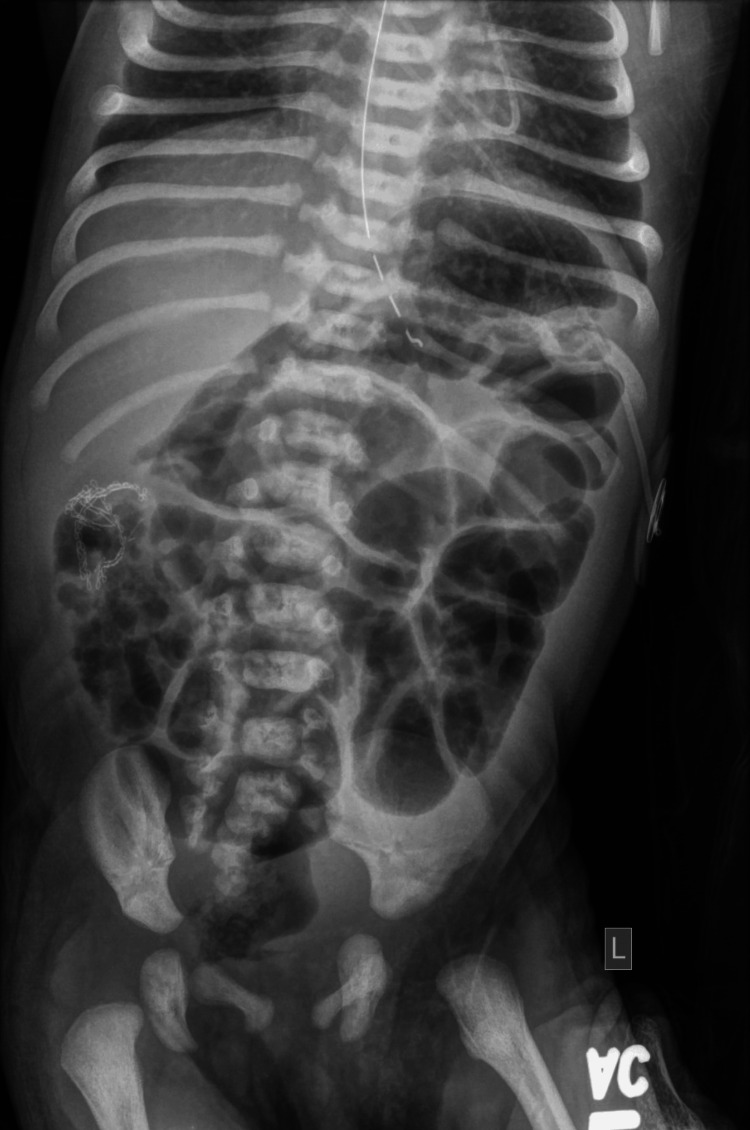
Abdominal radiograph shows the status post-surgical repair of ileal atresia with a relatively normal bowel gas pattern, which is now non-obstructive.
